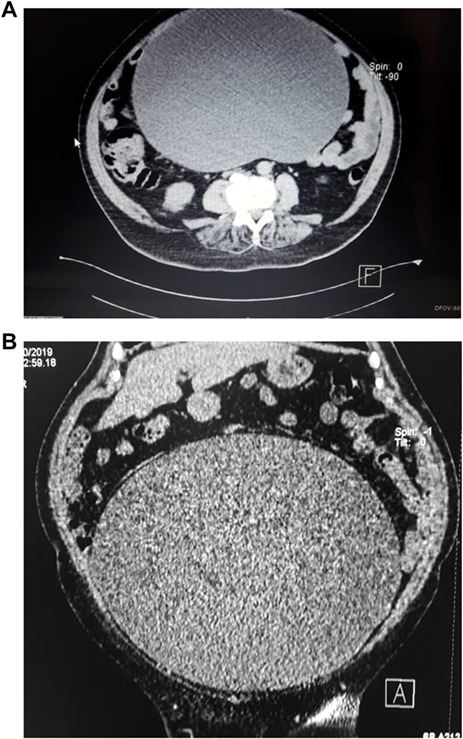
(A) CT scan, show giant cystic mass. (B) CT scan, showing ovarian tumor filling the lower abdomen.
